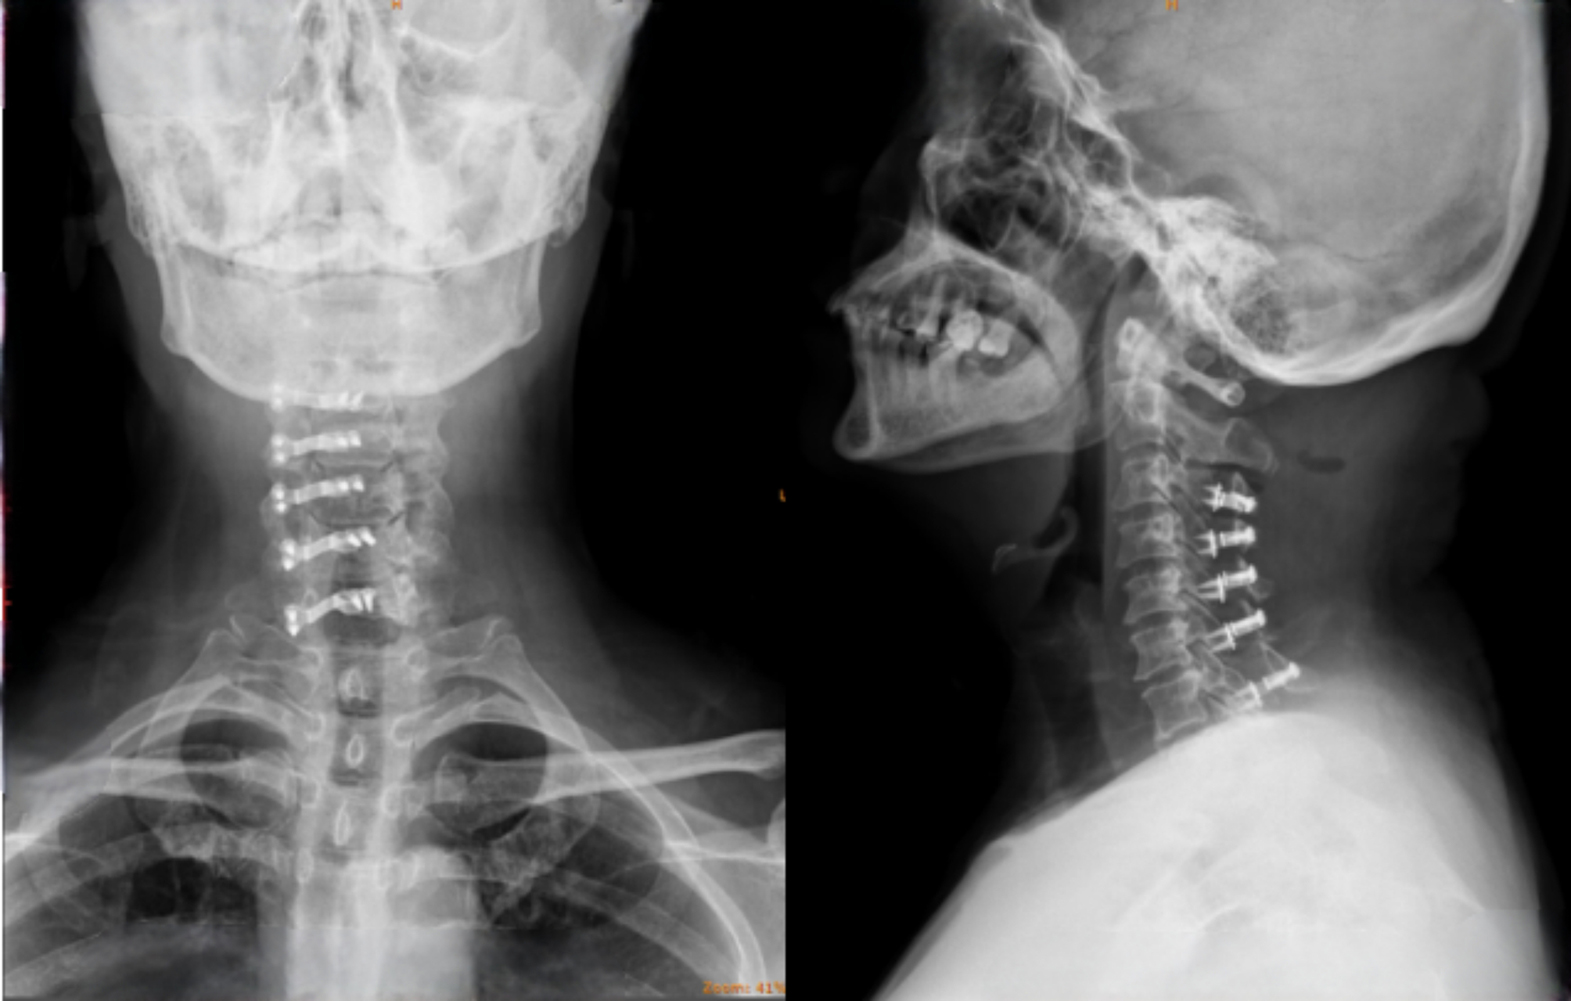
Postoperative anteroposterior X-ray and lateral X-ray showed good positioning of the screws and plates
